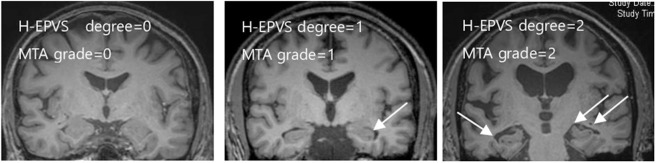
Examples of the degree of H-EPVS and MTA scale score. The three MRI images include each degree of H-EPVS and MTA scale score. White arrows point examples of H-EPVS. H-EPVS, hippocampal enlarged perivascular spaces; MTA, medial temporal atrophy.
